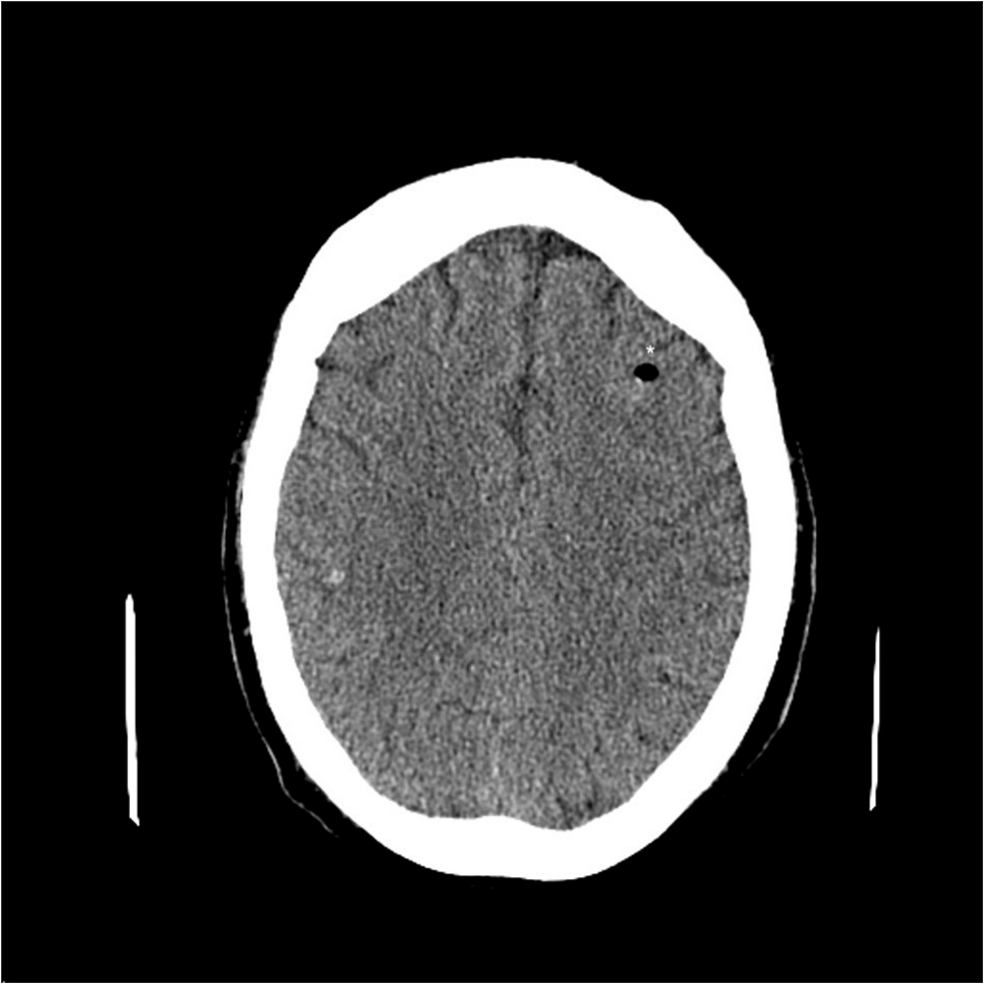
Computed tomography scan of the head showing hyperdense foci near gray-white junction in the left frontal and right parietal lobes with left frontal focus of pneumocephalus (noted as an asterisk “*”), highly suspicious for septic emboli.
